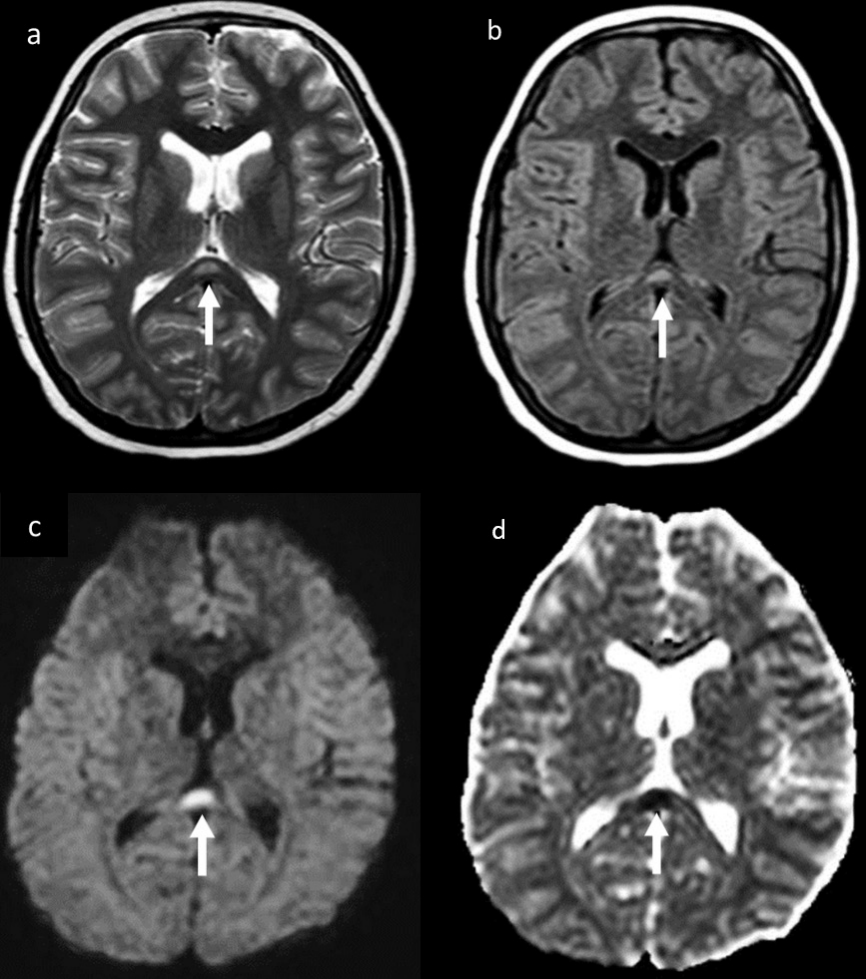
Cranial MRI of a 14-year-old boy who presented with multisystem inflammatory syndrome in children associated with SARS-CoV-2, with symptom cerebellar ataxia. A focal area of hyperintensity in the splenium of the corpus callosum on axial *T*_2_WI (a) and FLAIR (b) images corresponding to a focal area of restricted diffusion on diffusion-weighted (c) and apparent diffusion coefficient images (d). It was interpreted as MERS. FLAIR, fluid attenuated inversion recovery; MERS, mild encephalopathy with reversible splenial lesion; *T*_2_WI, *T*_2_ weighted imaging.
